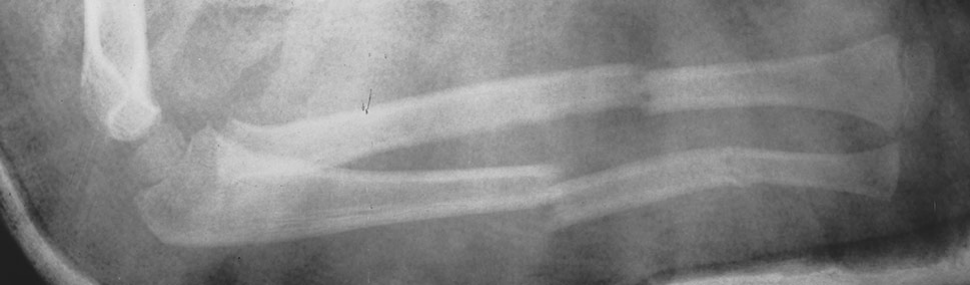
Radiograph of a 6-year-old boy with a type IV segmental injury of the ulna associated with a single diaphyseal fracture of the radius
